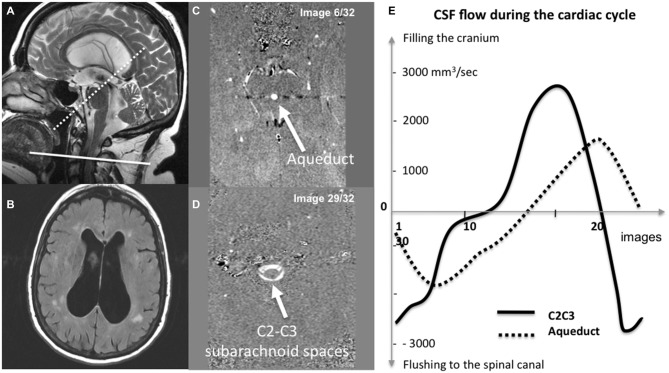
Quantification of cerebrospinal fluid (CSF) dynamics. A sagittal T2 weighted image (A) was used to select the phase-contrast magnetic resonance imaging (PCMRI) acquisition planes in patients with chronic hydrocephalus (CH) and ventricular dilation on the axial T2-weighted FLAIR image (B). Images (C,D) respectively show CSF flowing through the cerebral aqueduct and through the cervical (C2-C3) subarachnoid spaces during the cardiac cycle (CC). Post-processing of these PCMRI data enables the CSF oscillations during the CC to be quantified (D). Integration of these curves over time yields the CSF stroke volume (SV) for the aqueduct (SV_aqu_) and for the spinal canal (SV_spine_). The CSF SV corresponds to the volume of CSF moving through the slice over the CC and is the primary descriptor of CSF dynamics (E).
